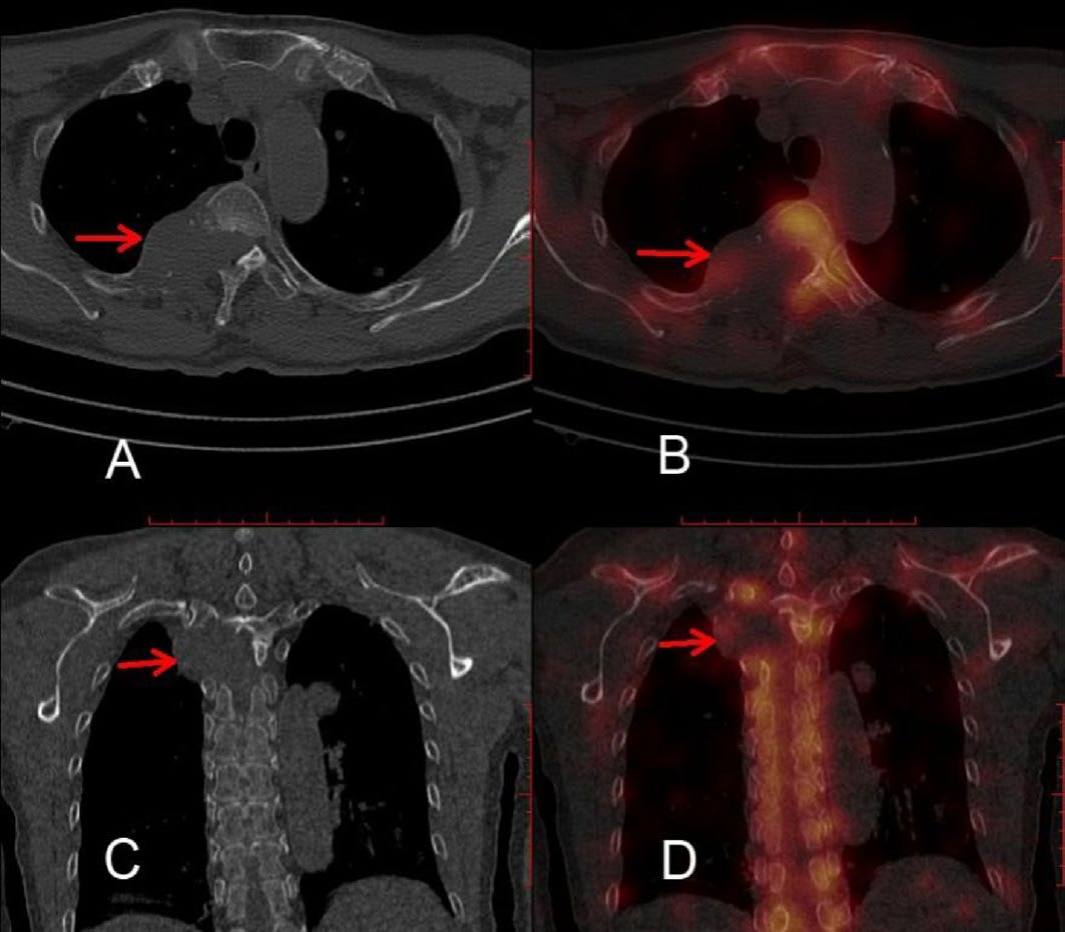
Presence of NRC on bone SPECT/CT scan.
